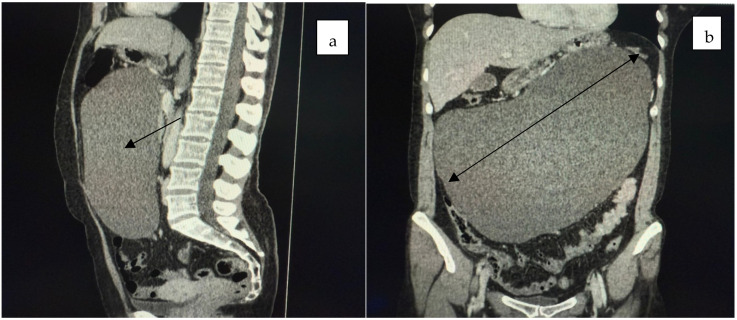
The sagittal ((a), black aroow) and axial ((b), black double arrow) CT scans of the abdomen and pelvis revealed a large tumor mass occupying the entire abdominal and pelvic cavity, causing partial compression of the stomach and exerting a mass effect on both the large and small intestines.
